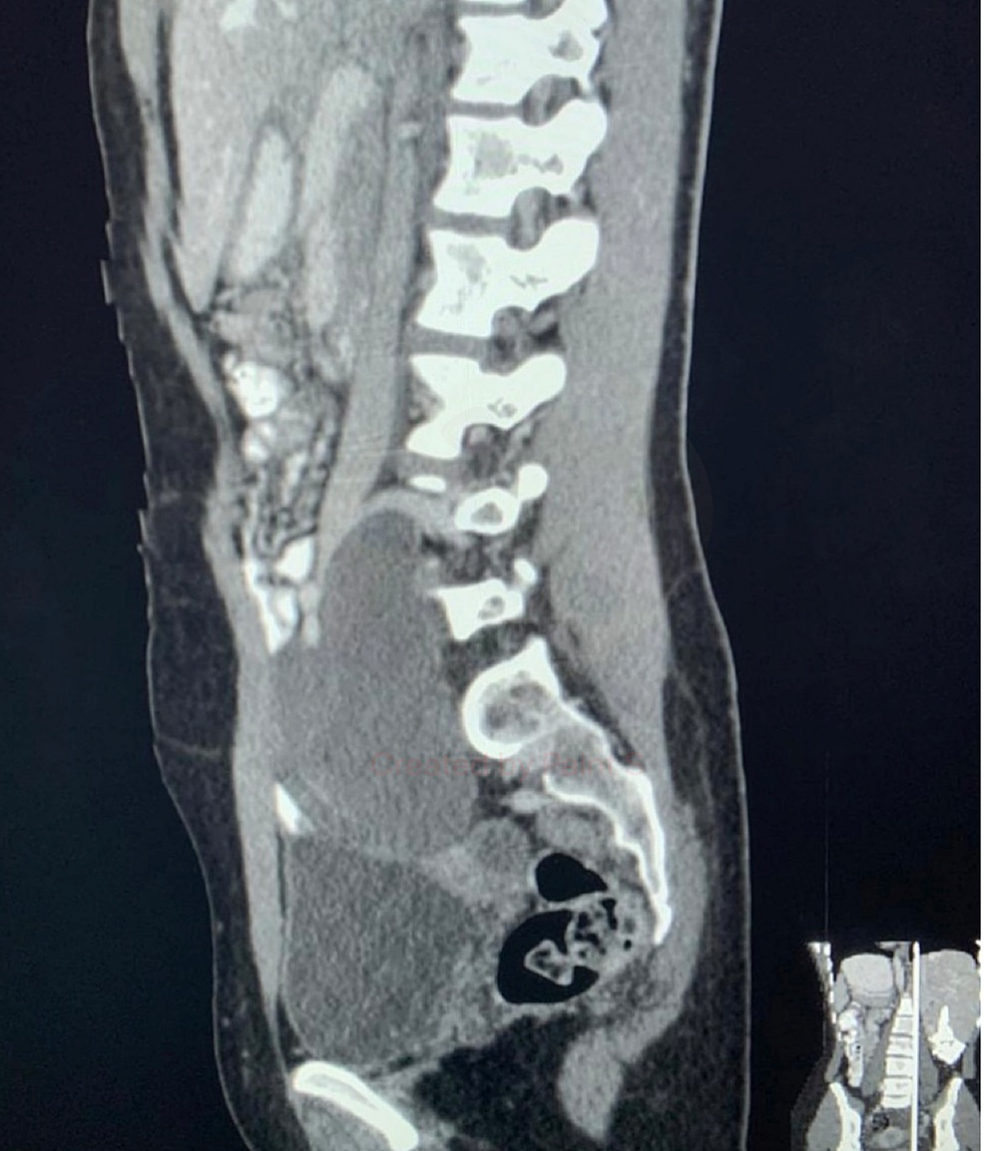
CT image (sagittal view) showing the retroperitoneal mass displacing the inferior vena cava.
